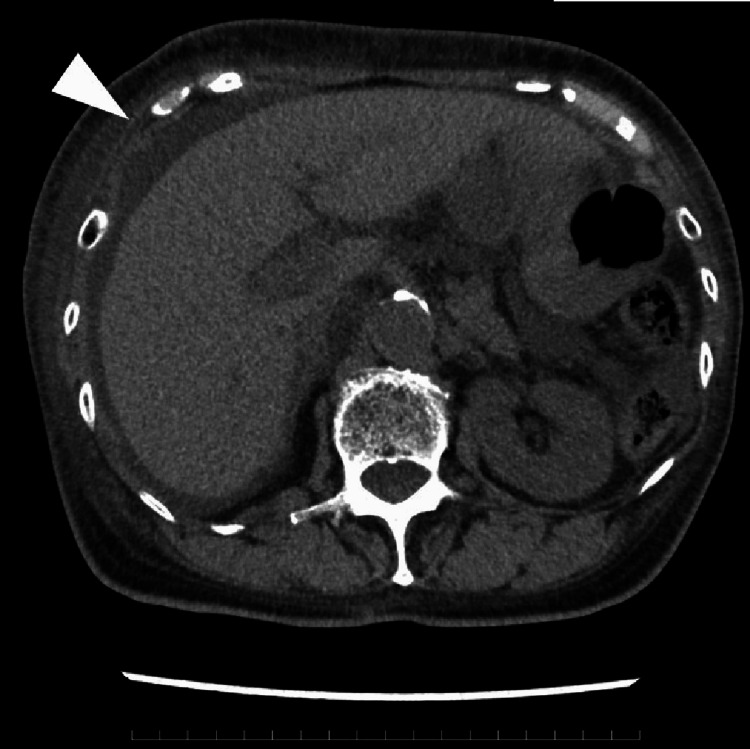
Ascites on abdominal computed tomography (in November 2024) A small amount of ascites (white arrow) were seen on the liver surface with increased pericardial fluid
